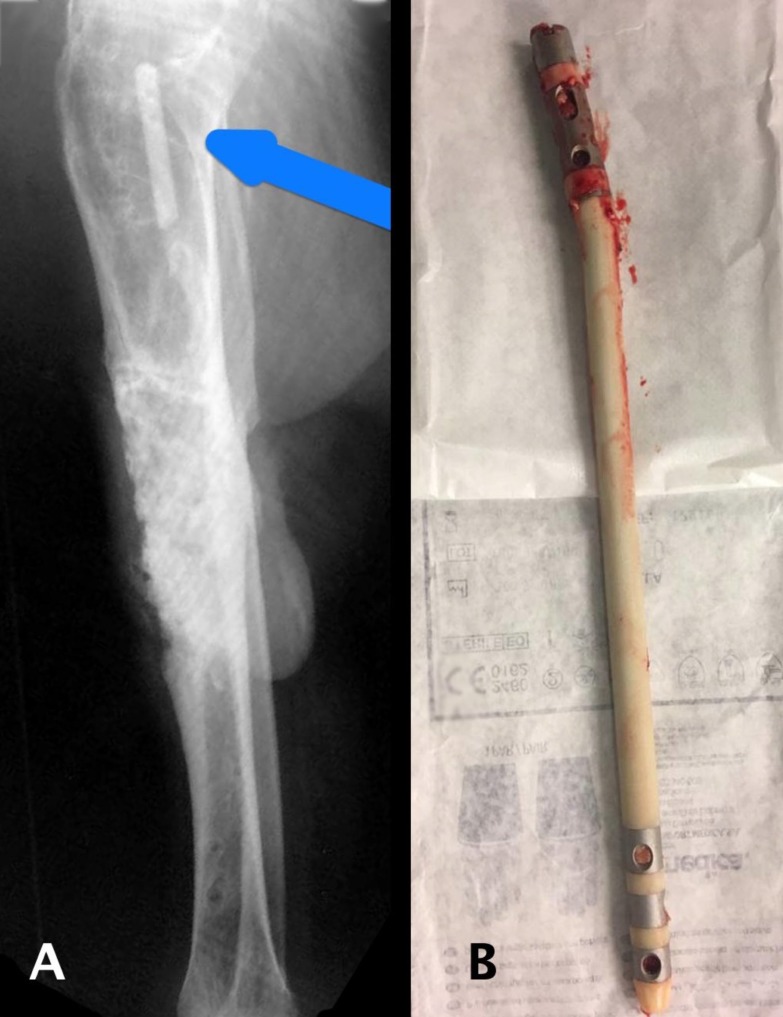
A: A lateral X-ray shows a broken piece of PMMA in the diaphysis of a tibia after a reconstructive process, indicated by the arrow. B: Image of a nail without mantle break after removal.
